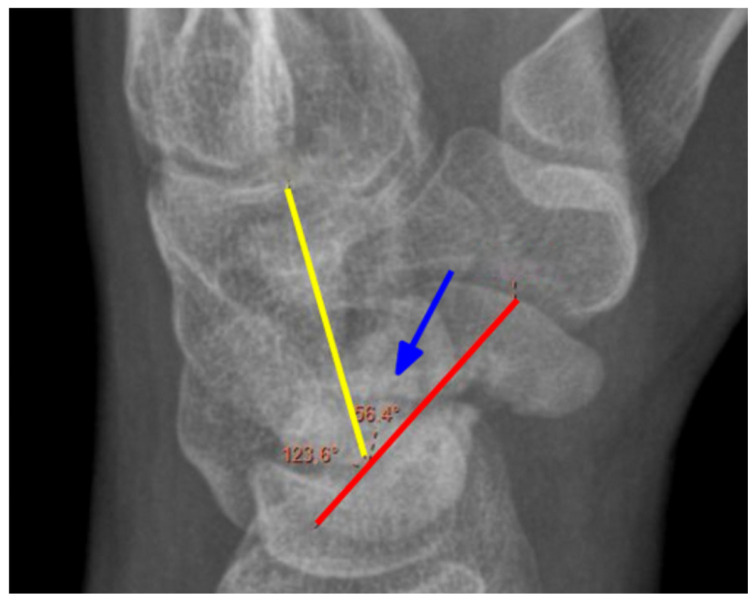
Measurement of the SCA angle. Blue arrow—angle, yellow line—the axis of the capitate, red line—the axis of the scaphoid. X-ray of the right hand, PA view.
